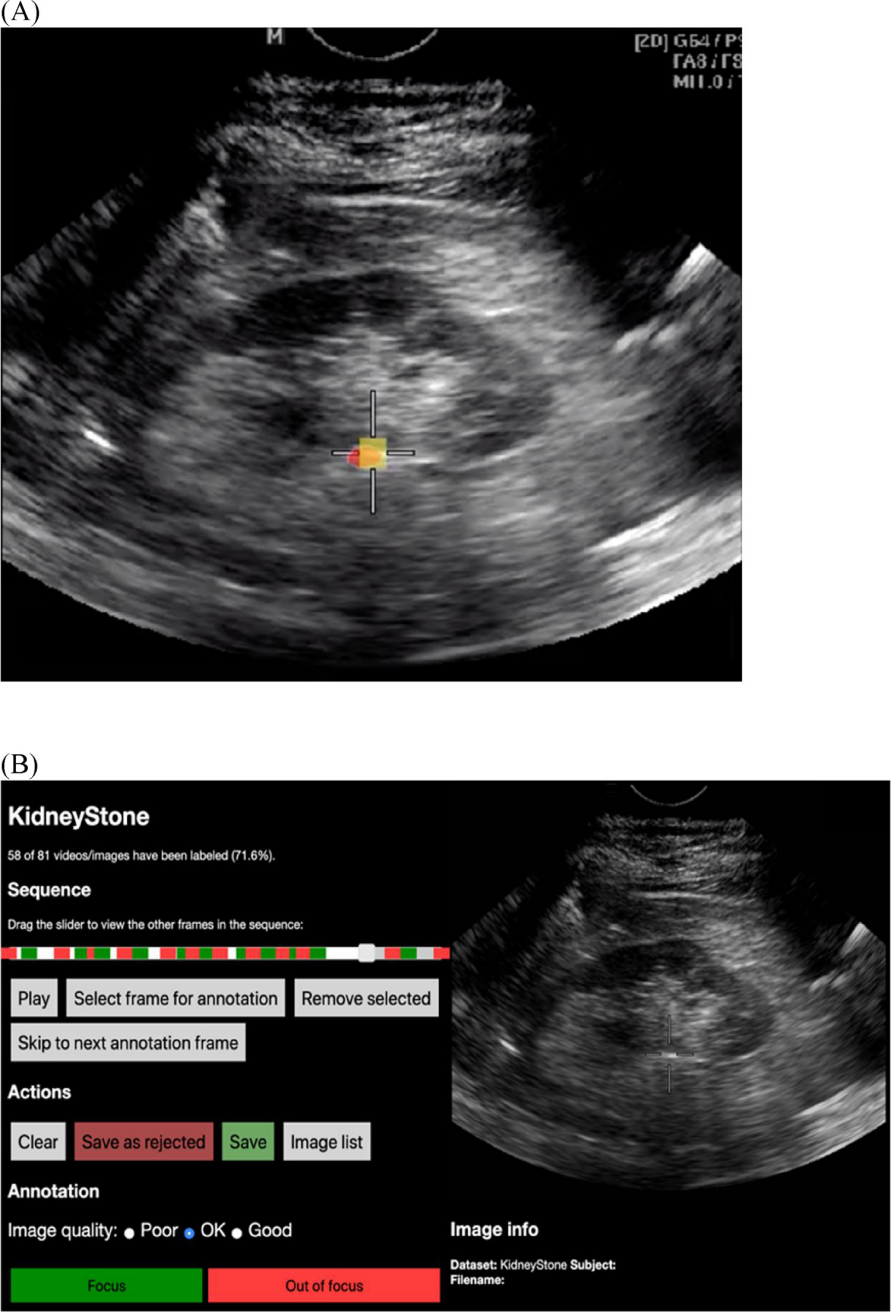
(A) Example of a frame for which the model reported that the stone was in focus, as ≥50% of the predicted stone (red) was within the focal zone (yellow). (B) Screenshot of the annotation tool. The ultrasound video with crosshairs is shown to the right, and a slider is used to go through the frames. To annotate a frame, the annotator clicks “Select frame for annotation” and chooses to label the frame as either “focus” or “out of focus”. If the stone is in focus for this frame, the annotator then continues the video and stops to label the first frame for which the stone goes out of focus. The frames between these two labels are automatically labeled “focus”. The green and red rectangles represent the frames labeled by the annotator.
